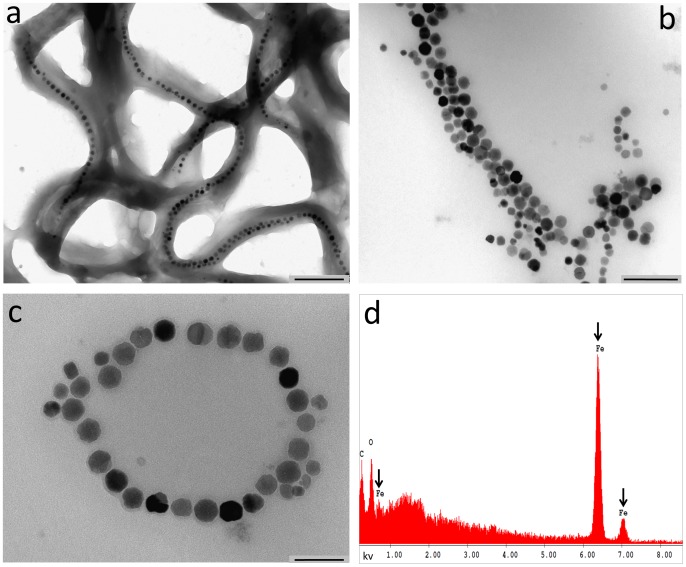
TEM of MNs. Panel a shows the organization of MNs in chains in the bacteria (scale bar, 500 nm). Panels b-c show that the typical conformation of chains is maintained after isolation of MNs. Scale bars: b>200 nm, c>100 nm. Panel d, X-ray microanalysis shows the iron content of the MNs.
